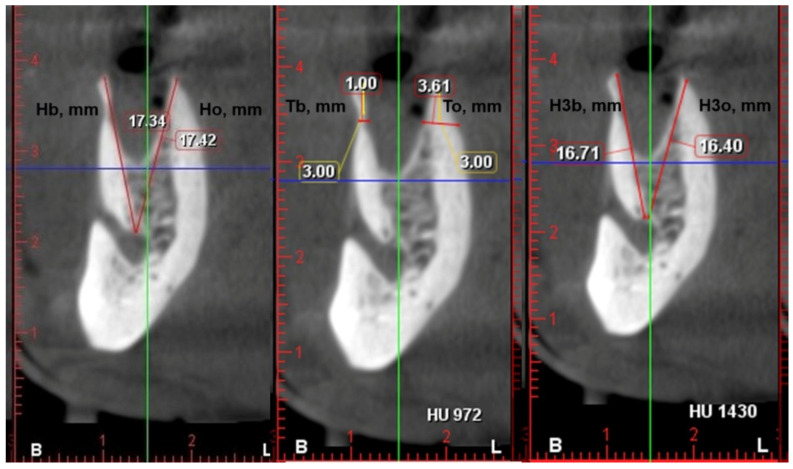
Measurement of the height and thickness of the socket plates using cone-beam computed tomography. Hb—buccal plate height on day 1; Ho—oral plate height on day 1; Tb—buccal plate thickness on day 1; To—oral plate thickness on day 1; H3b—buccal plate height 3 months later; H3o—oral plate height 3 months later.
